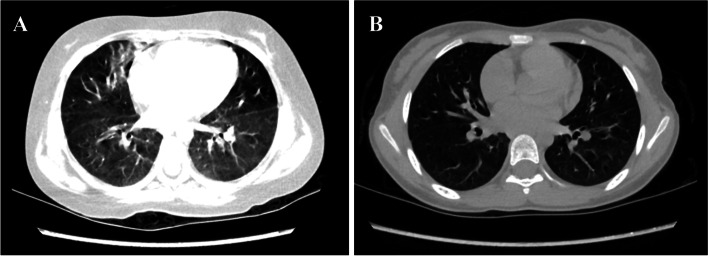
Comparison of chest HRCT images before (A) and after (B) treatment of CYC. A Diffused interstitial changes in both lungs. The brightness of the two lungs is uneven, extensive ground glass shadows, fiber strips can be seen. B The uneven brightness of both lungs improved, fiber strips and patchy shadows reduced compared with before
